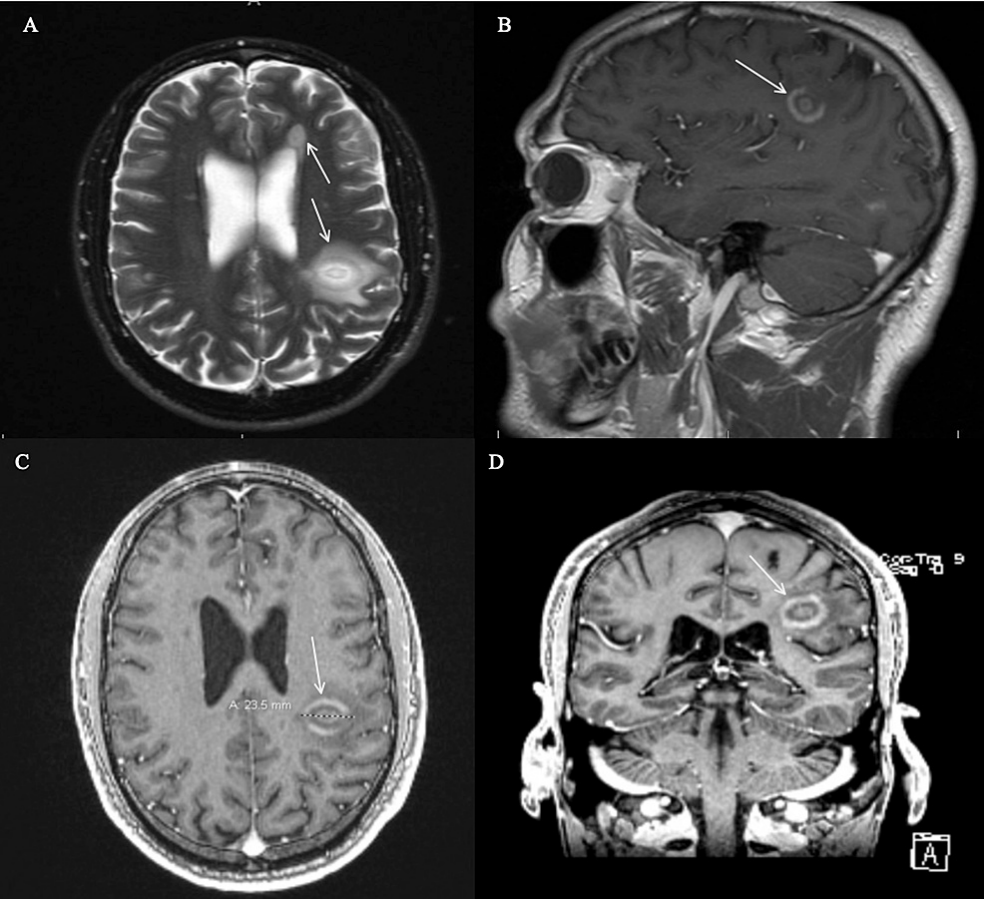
(A-D): MRI brain with and without contrast. Figure 1A shows numerous T2-hyperintense white matter lesions within the subcortical periventricular deep white matter and corpus callosum. Figure 1B shows a concentric bullseye area of T1 isointense and low-signal intensity seen in the left parietal lobe with surrounding vasogenic edema and enhancement measuring 2.1 cm X 1.8 cm X 1.6 cm. Figures 1C and 1D show a smaller enhancing lesion in the left posterior temporal lobe, demonstrating wispy enhancement from an axial and coronal view. Several lesions demonstrate low T1-signal intensity/black holes. There was no intracranial hemorrhage, mass lesion, or acute infarct. There was no extra-axial fluid collection or hydrocephalus.
